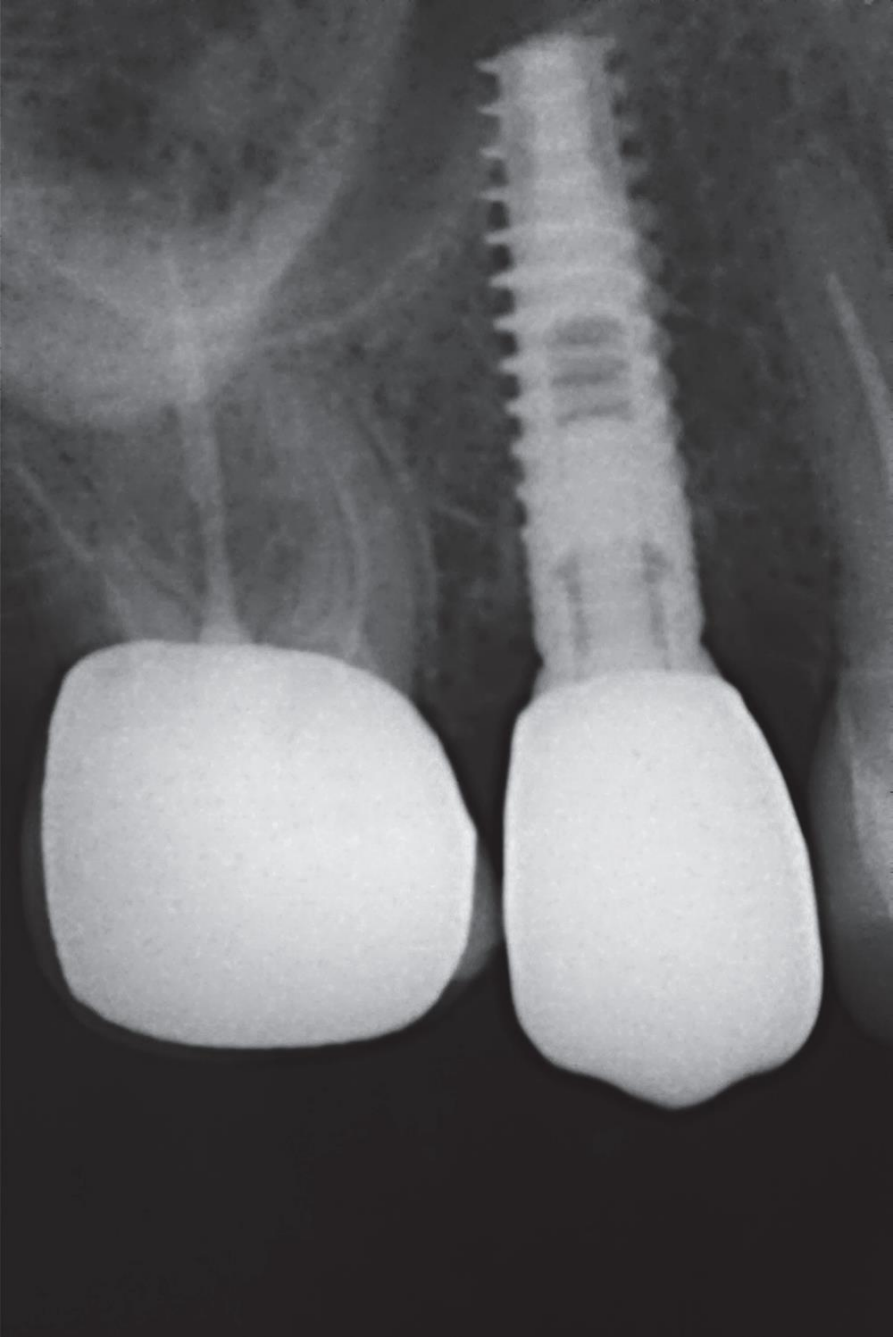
Postoperative radiographic image at the 1‐year follow‐up confirming maintained crestal bone level and accurate prosthesis fit.
